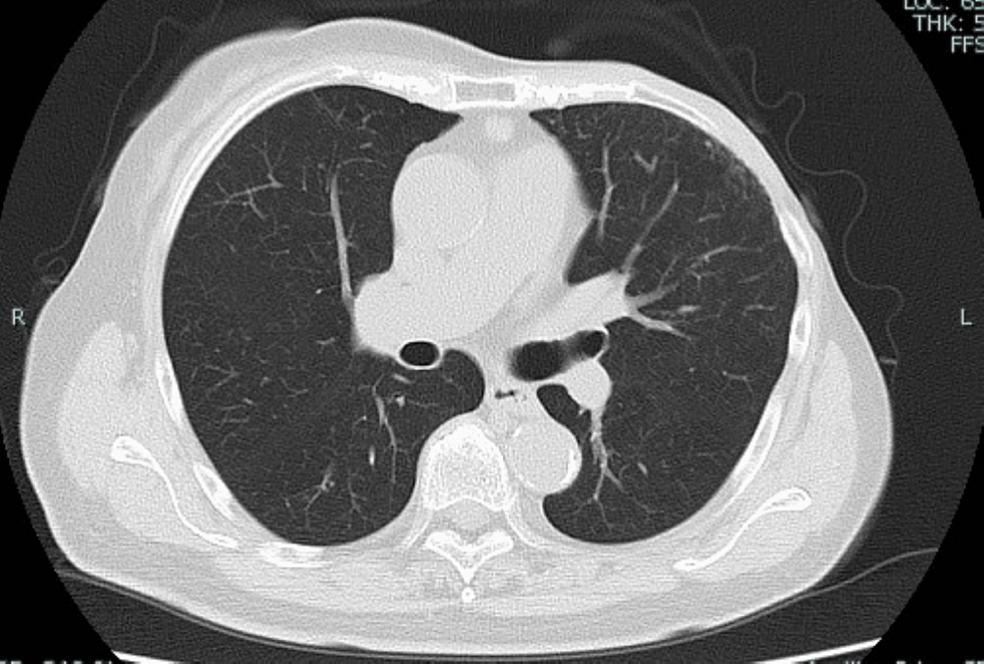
Chest CT (six months after onset) It showed improvement without pulmonary fibrosis.
